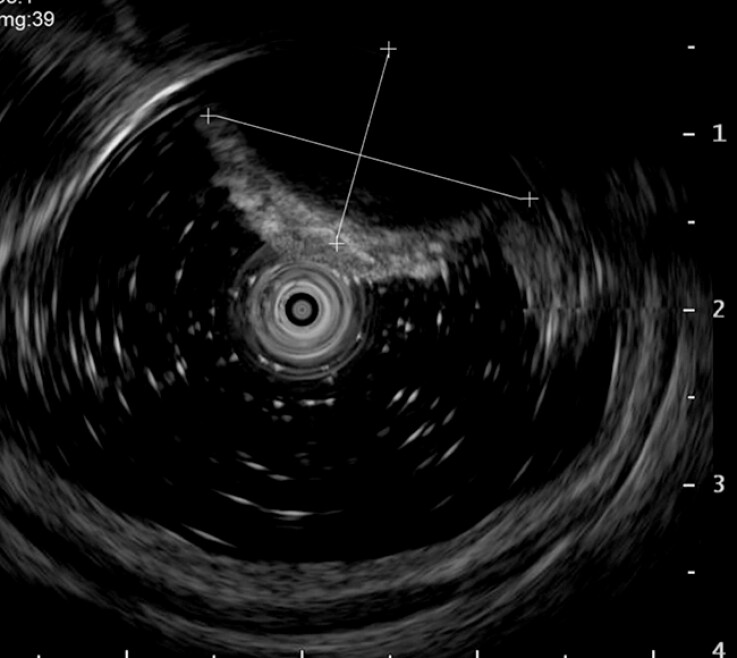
Transrectal endoscopic ultrasound showing a 4-cm hypoechoic mass.
